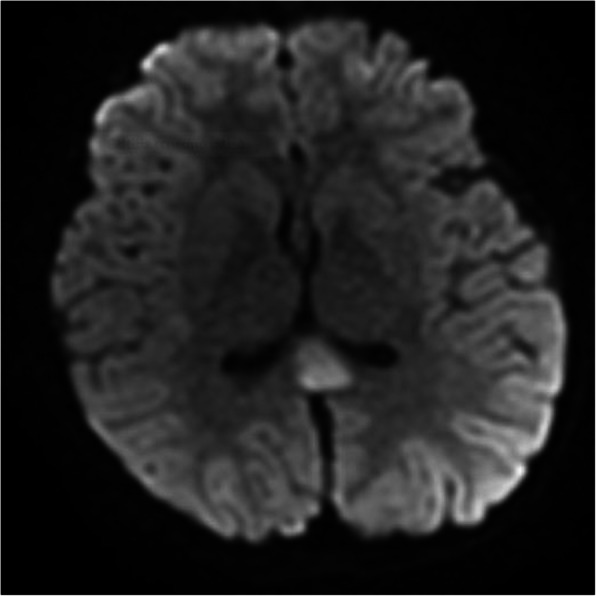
MRI DWI: lesion of the splenium of corpus callosum (transversal section)
